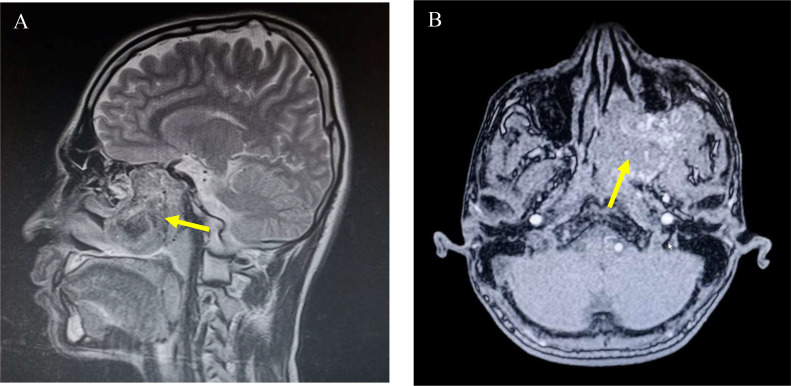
(A) Sagittal T2-weighted image: Demonstrates a large, heterogeneously hyperintense mass occupying the nasopharynx and extending superiorly. The yellow arrow highlights the extensive nature of the lesion. (B) Axial T1-weighted postcontrast image: Shows intense heterogeneous enhancement of the mass. The yellow arrow points to multiple punctate and curvilinear “flow voids” (dark signal spots), which represent high-velocity blood flow within the tumor’s rich vascular network, a characteristic feature of Juvenile Nasopharyngeal Angiofibroma (JNA).
